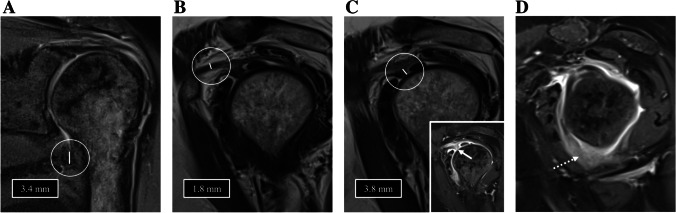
Images of the right shoulder of a 58-year-old female with clinical signs of ACS. A Coronal PD-weighted image shows a non-thickened capsule at the axillary recess with predominantly regular signal intensity. The CHL (B) (1.8 mm) as well as the capsule at the rotatory interval (C) are not thickened in T2-weighted sagittal imaging. However, the rotatory interval (C, arrow in small image on bottom right) and the axillary recess (D, dotted arrow) show distinct contrast uptake in T1-weighted fat-saturated contrast-enhanced sagittal imaging indicating the presence of ACS
